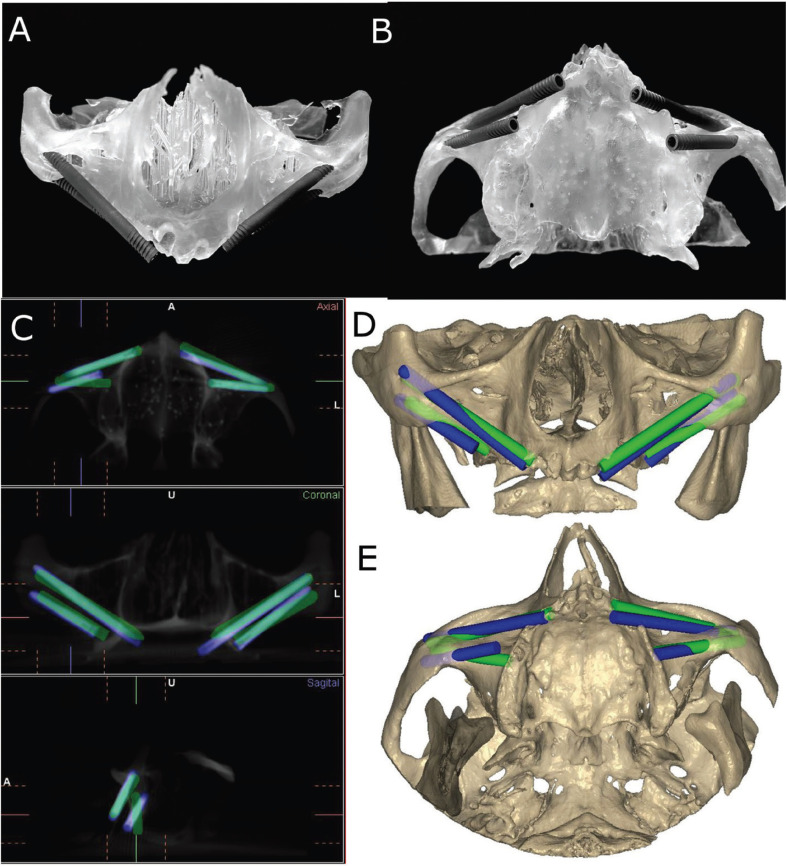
(A) Front and (B) bottom view of the zygomatic dental implants placed on the experimental model. (C) CBCT images, (D) front and (E) bottom view of the measurement procedure between the preoperative planning (green cylinders) and postoperative zygomatic dental implant placement (blue cylinders).
